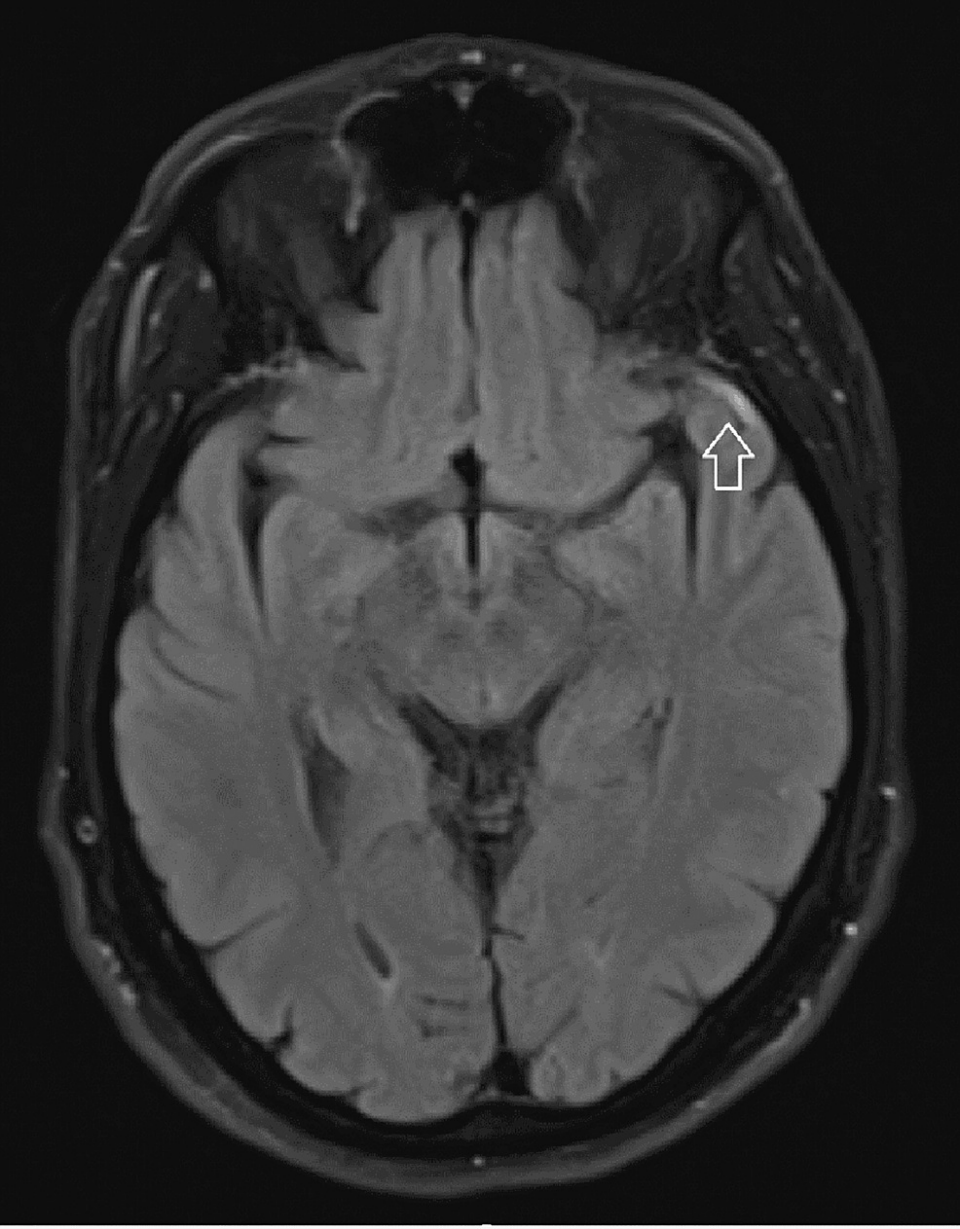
MRI-FLAIR (axial view) with hyperintensity signal due to thrombosed cerebral vein at the temporal cortex convexity MRI: magnetic resonance imaging; FLAIR: fluid-attenuated inversion recovery
